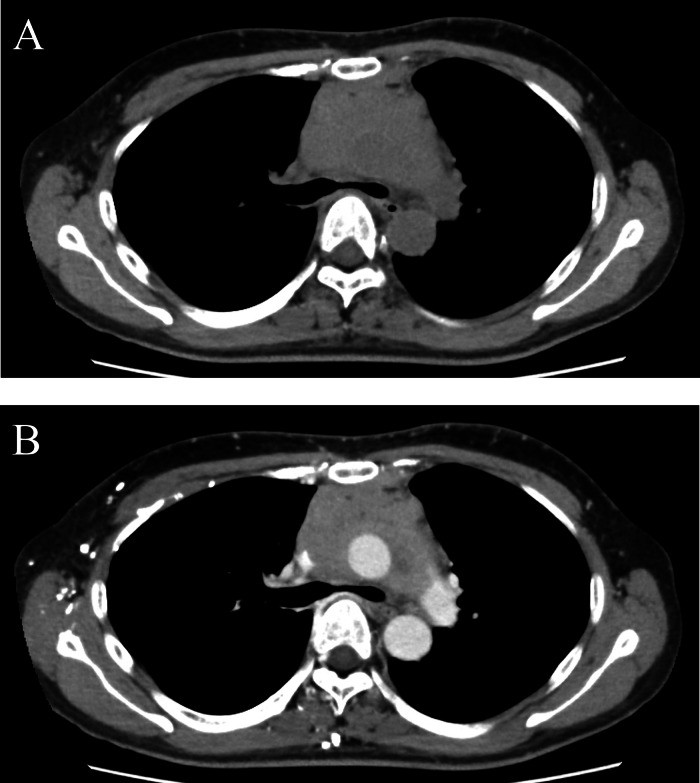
Axial images of plain CT scan(A), contrast-enhanced CT scan(B) in the second case Axial CT scan images showing a poorly defined soft tissue mass in the mediastinum causing left bronchus narrowing. The mass presents homogenous contrast enhancement.
